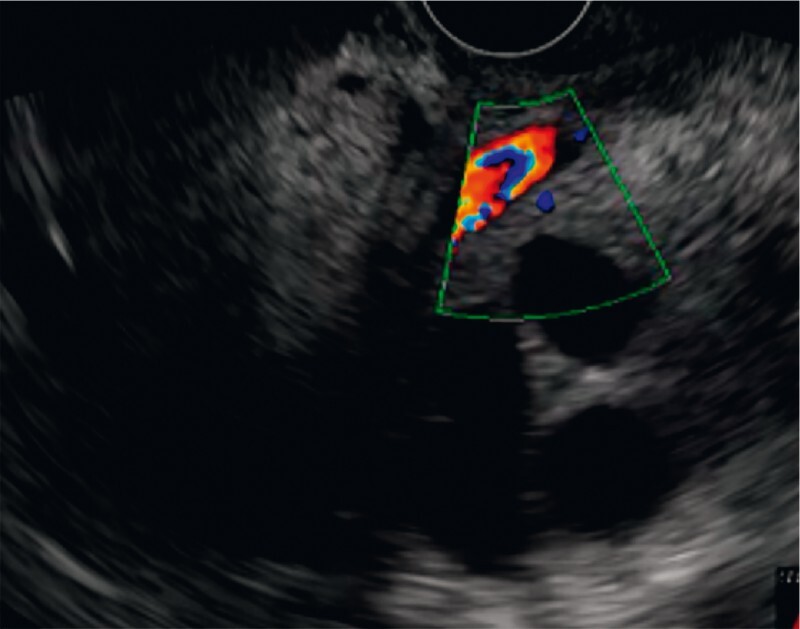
Endosonography image from the duodenal bulb showing tumoral infiltration and interposed vessels, excluding the possibility of performing a choledochoduodenostomy.
